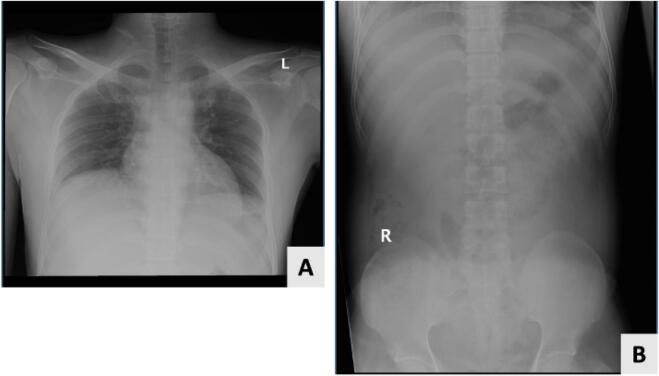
Plain radiographs failed to identify the foreign body. A: Plain chest X-ray. B: Plain abdominal X-ray.
